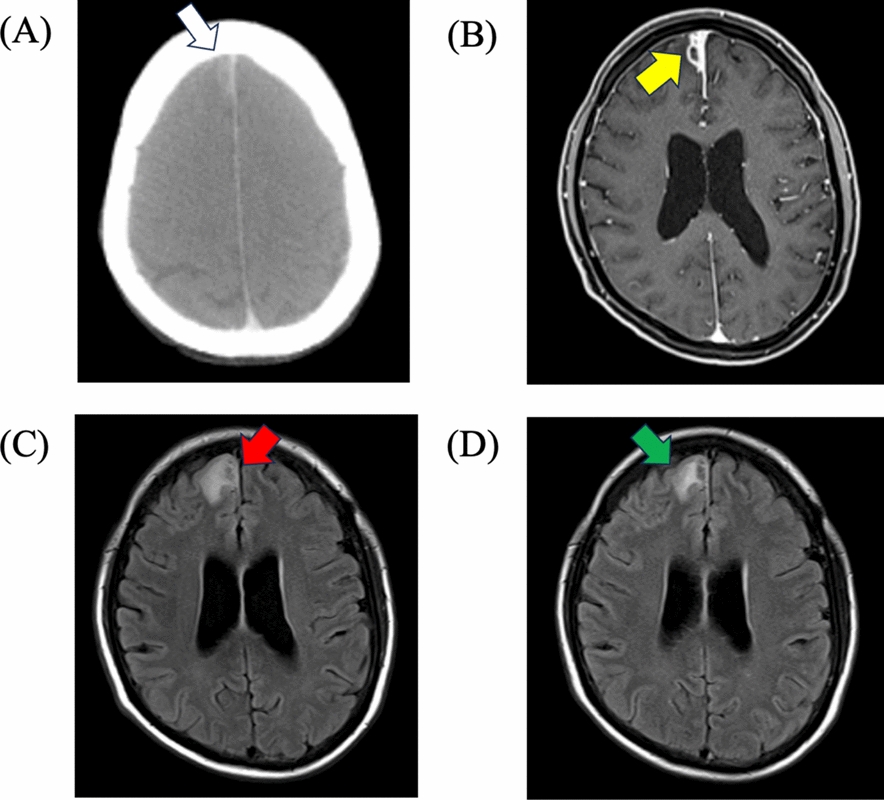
Axial imaging findings. A Brain computed tomography image shows a hyperdense lesion adjacent to the right falx cerebri (white arrow). B Contrast-enhanced T1-weighted magnetic resonance image shows cortical and parenchymal lesions in the right frontal lobe (yellow allow). C T2-weighed magnetic resonance image shows hyperintense edema surrounding the right side of the falx cerebri (red arrow). D Follow-up T2-weighted magnetic resonance image on day 14 showing reduced perilesional edema (green arrow)
